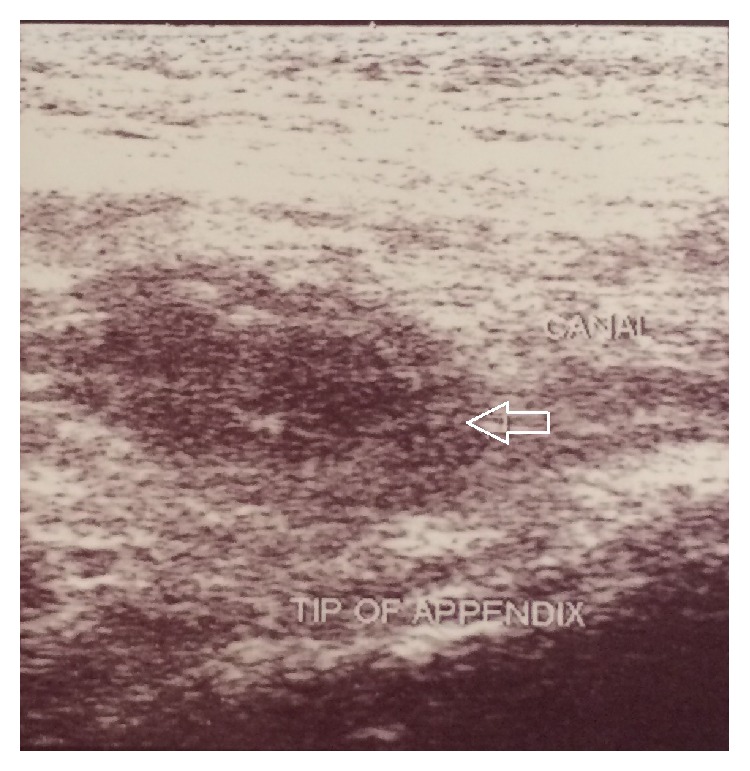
Ultrasound study shows right inguinal canal with the tip of appendix herniated into the canal (white arrow).
